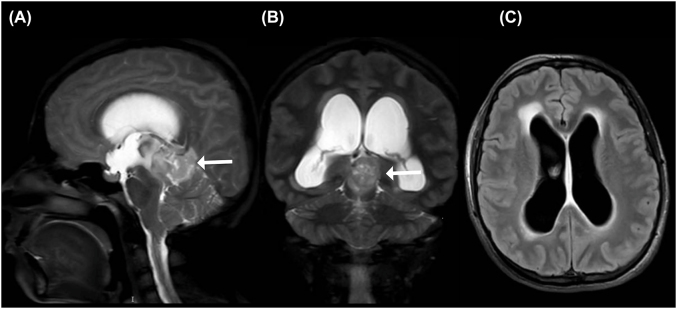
Brain magnetic resonance imaging. (A, B) Mass in the pineal projection area with a heterogeneous signal with areas of intralesional necrosis (white arrow), obliteration of the supra and infratentorial subarachnoid space with a compressive effect on the midbrain, bridge and medulla. (C) Changes suggestive of ventriculomegaly, dilation of the supratentorial ventricular system with transependymal migration.
